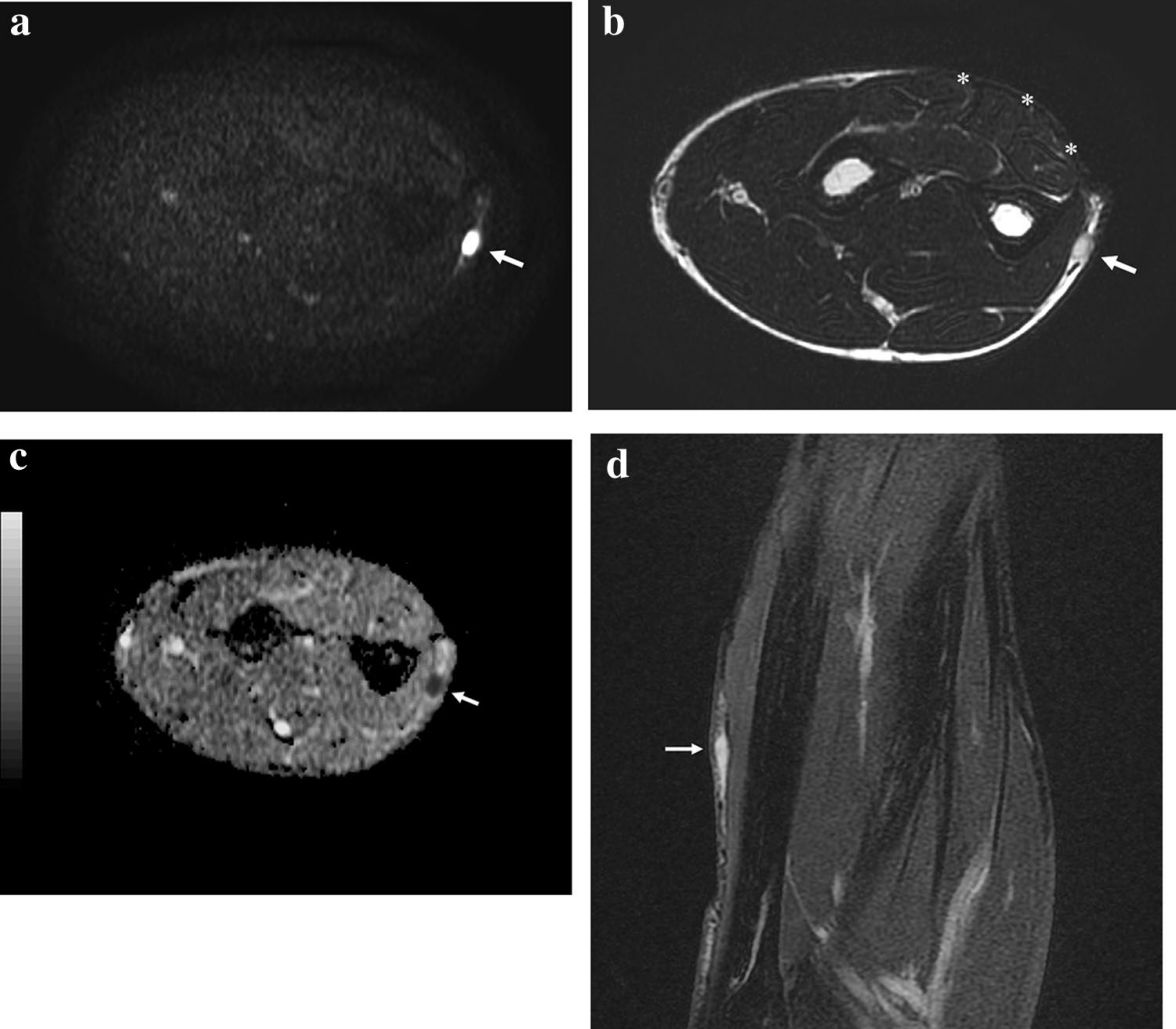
Example of the greater conspicuity of a lesion on DWI (*b* = 1000) compared to T2w and ADC map images. Fifty-one-year-old man with recurrent myxofibrosarcoma in the left forearm (a, b, c, d). Asterisks indicate scar tissue. a Axial DWI shows a small marked hyperintense nodule (*arrow*) in the subcutaneous fat with greater conspicuity compared to b T2w image, c ADC map, and fat-sat T2w image (d). Note the fibrous post-surgical scar tissue (*asterisks*) depicted on the T2w image
